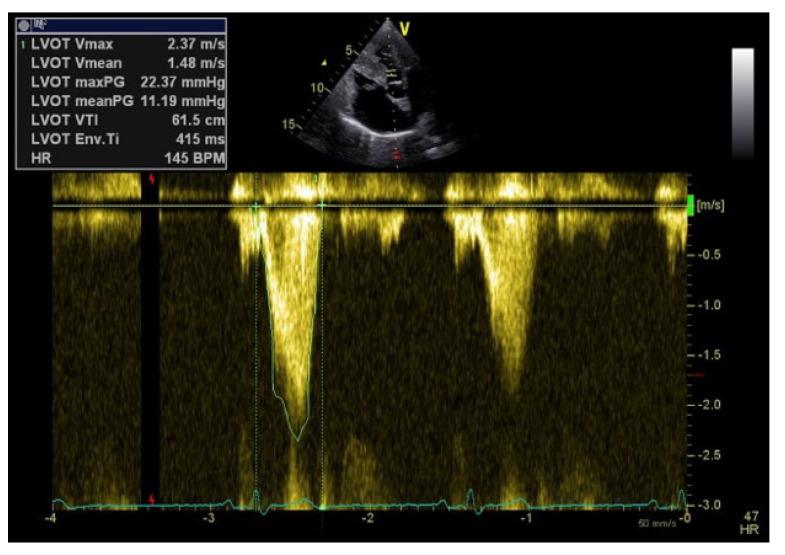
Typical morphological appearance of the Doppler signal “dagger−shaped” and late peaking curve in left ventricular outflow tract obstruction.
